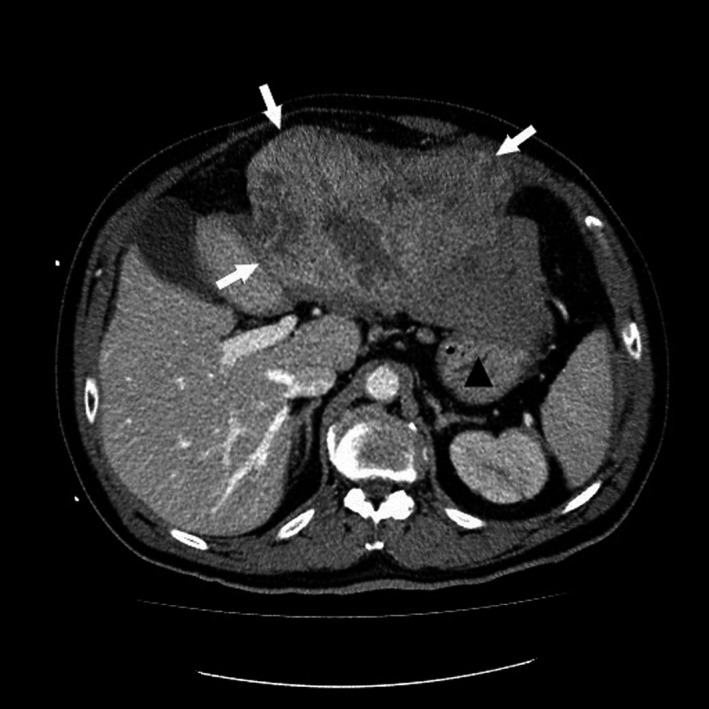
A CT scan in 2016 showed a tumor‐altered left part of the liver (white arrows), with an 8‐cm‐diameter HCC. Note the massive hemoperitoneum (black triangle). CT, computed tomography; HCC, hepatocellular carcinoma
